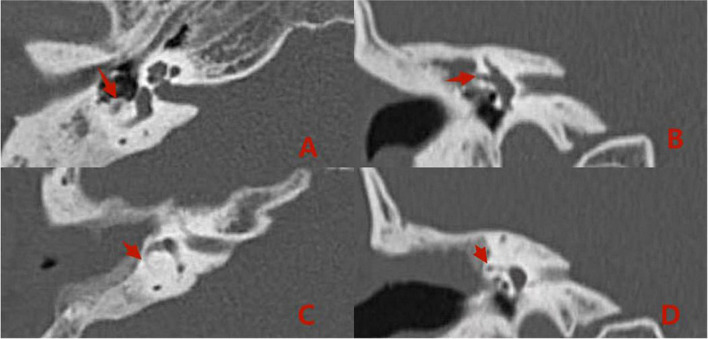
Computed tomography (CT) images acquired (A,B) preoperatively from a patient with type I labyrinthine fistula. Preoperative (A,B): The right cholesteatoma is adjacent to bone resorption, the boundary with the external semicircular canal is unclear, and the shape of the external semicircular canal is acceptable (the red arrows). Postoperative (C,D): The cholesteatoma has been removed, the shape of the lateral semicircular canal is acceptable, and intraoperative fillers are applied to fill the external semicircular canal (the red arrows).
